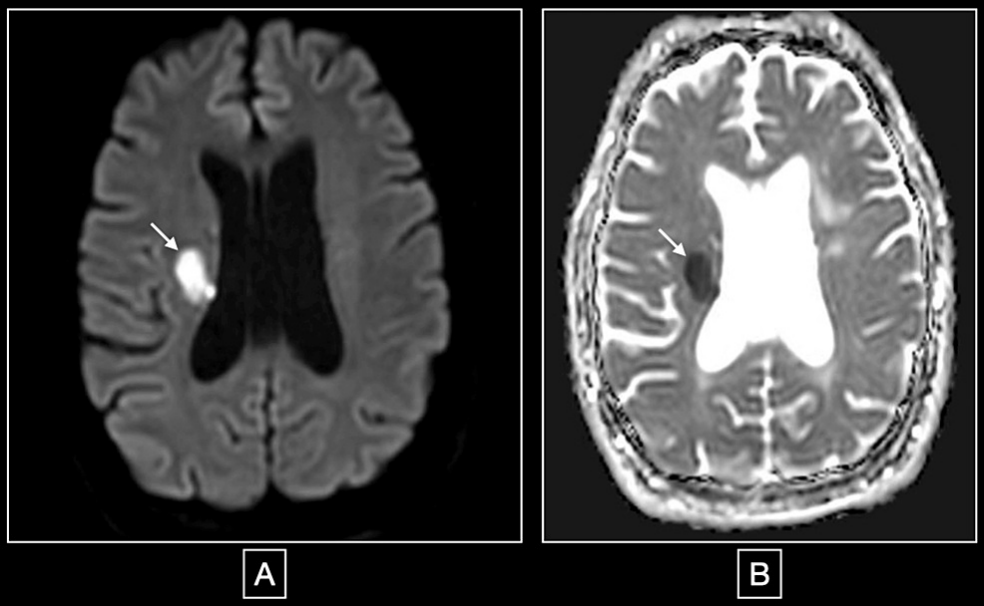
DWI and ADC sequences showing an acute insular stroke Figure A (image on the left) is the DWI sequence, and figure B (image on the right) is the ADC sequence of the MRI brain, and both show an acute right stroke of the subcortical insular lobe extending into the corona radiata (signaled by a white arrow). ADC: apparent diffusion coefficient; DWI: diffuse weighted image
